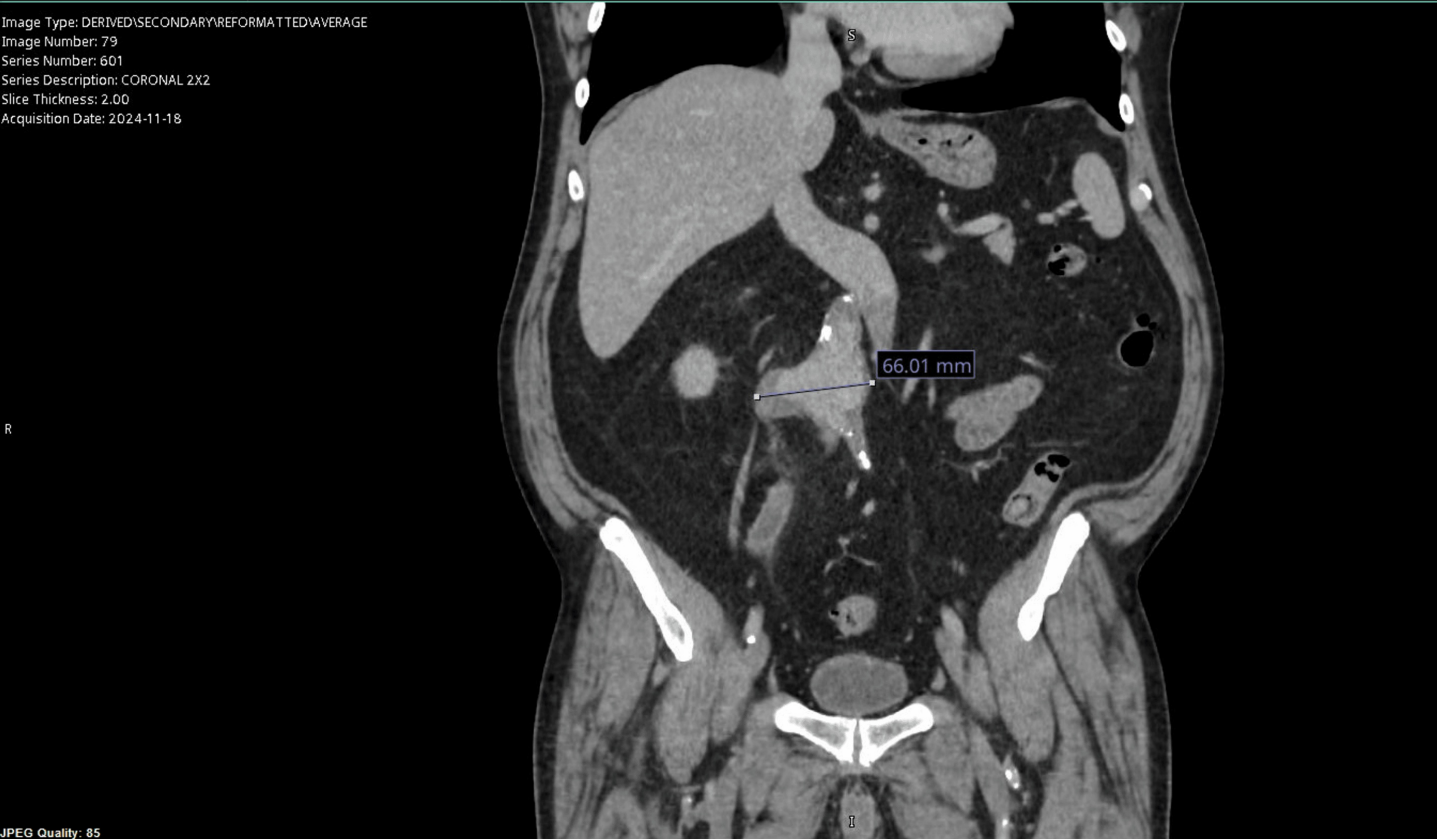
The patient’s abdominal CT scan, showing evidence of the abdominal aortic aneurysm with a saccular component, measuring >6 cm
